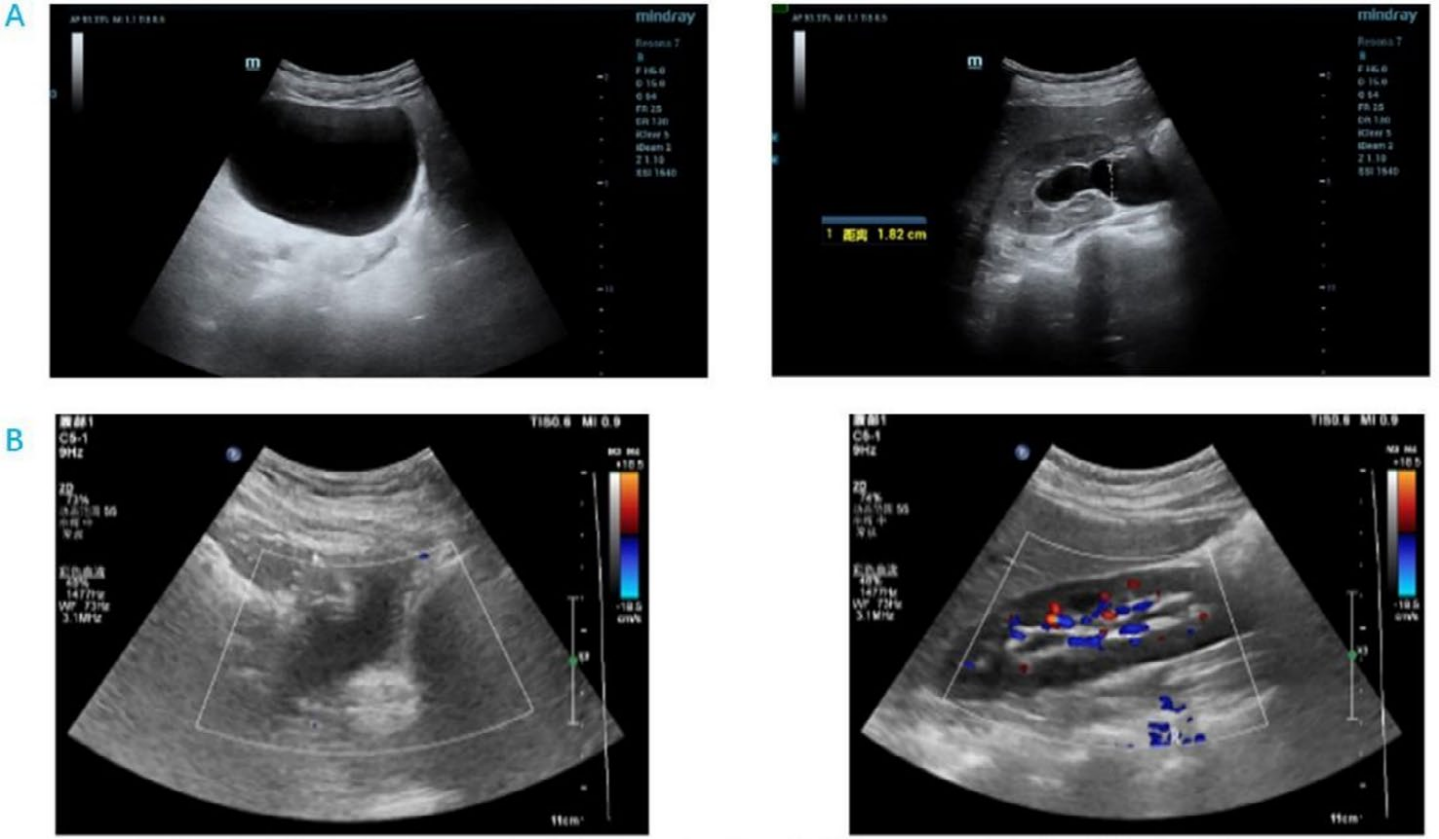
Ultrasound of the urinary tract before starting PRP treatment (A), Ultrasound of the urinary tract after completion of PRP treatment (B).
